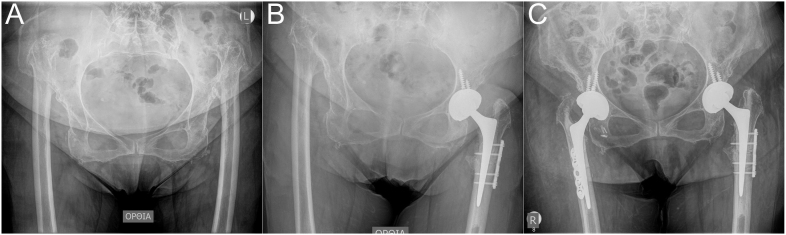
Preoperative (A) and postoperative (B and C) radiographs of bilateral Hartofilakidis C2/Crowe IV developmental dysplasia of the hip managed with hybrid total hip arthroplasty using cemented Exeter stems combined with transverse subtrochanteric osteotomy at two separate stages. The osteotomy site was stabilised using a dynamic compression plate and screws.
